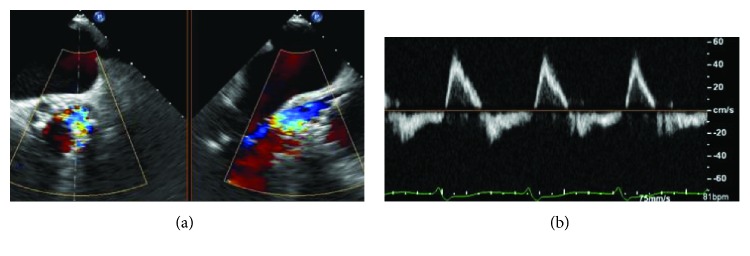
(a) Transoesophageal echocardiography showing a severe paravalvular leak after deployment of a CoreValve Evolut R 34 mm. (b) Pulsed wave Doppler in the descending aorta showing the presence of a consistent holodiastolic retrograde flow.
